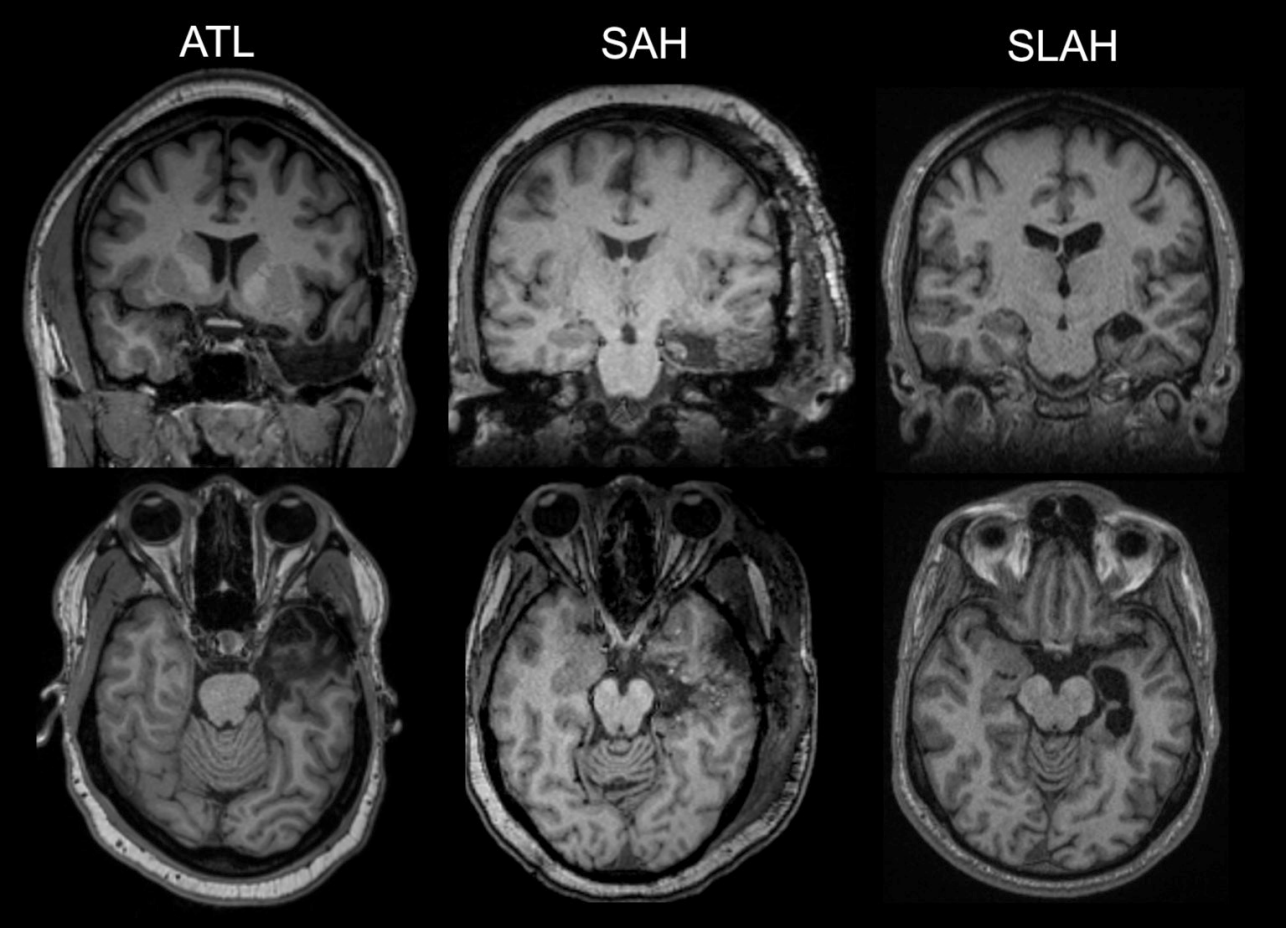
Comparison of selective laser ablation of amygdalohippocampal complex and open resections of the anterior temporal lobe. The first MRI image in each row represents an anterior temporal lobectomy (ATL). The second MRI image is a transcortical selective amygdalohippocampectomy and parahippocampectomy (SAH). The third images on the far right depict a stereotactic laser amygdalohippocampotomy (SLAH), which completely spares the lateral and anterior temporal structures. The MRI images in the top row are from a coronal viewpoint and those in the bottom review are an axial representation. All images are presented using standard neuroradiological convention. (left side of image represents right hemisphere of brain and vice versa). All represent left TL procedures.
